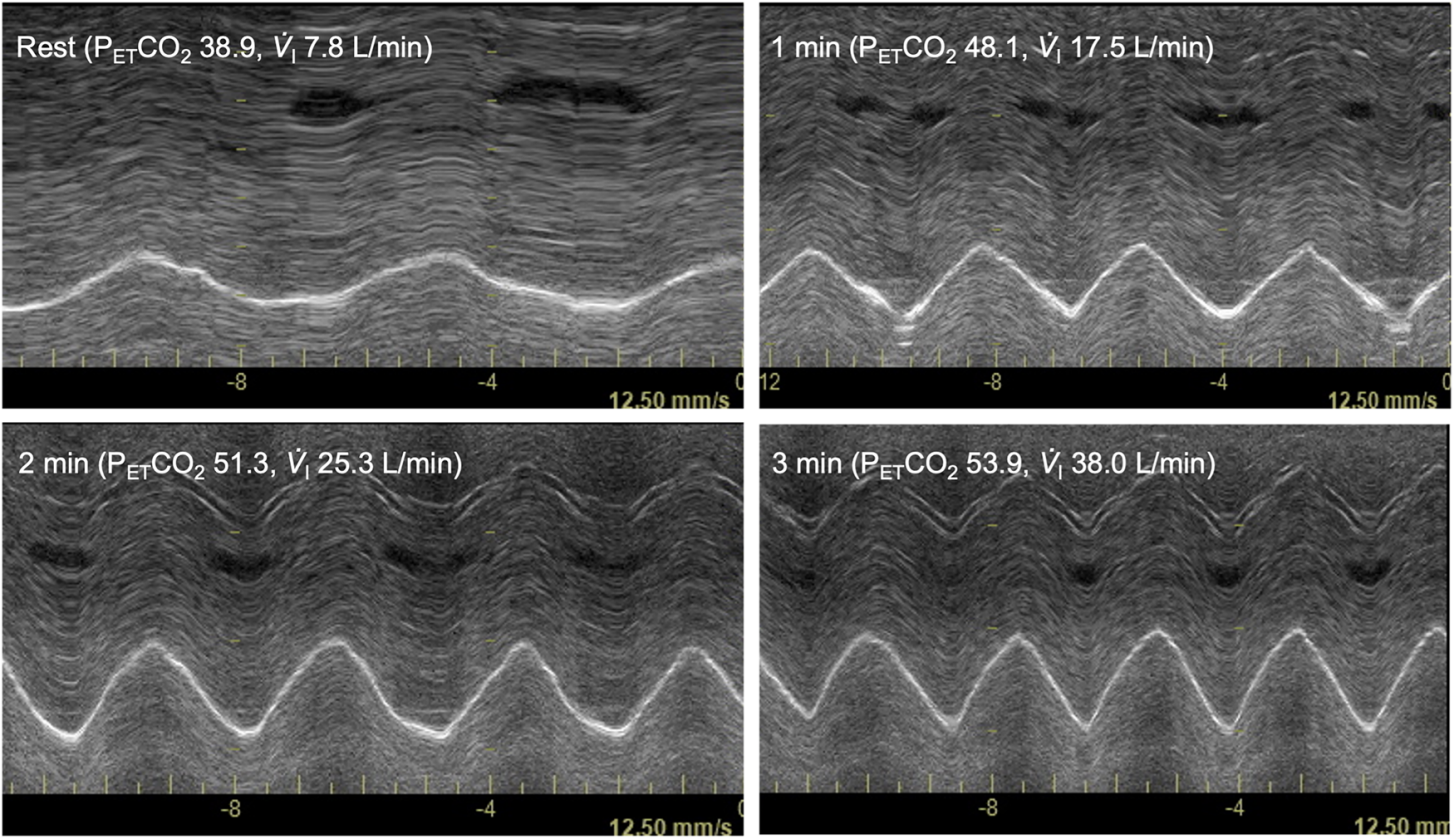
Representative anatomical motion‐mode (AM‐mode) ultrasound images of the right crural hemidiaphragm during quiet breathing (rest) and after 1, 2 and 3 min of CO2 rebreathing in a female participant. Diaphragm excursion increased progressively with rising ventilation (1.81 cm at rest; 4.89 cm at 3 min). Axis scales are identical across panels. Abbreviations: $P_{\mathrm{ET},CO_{2}}$, end‐tidal partial pressure of CO_2_; $\dot{V}$
